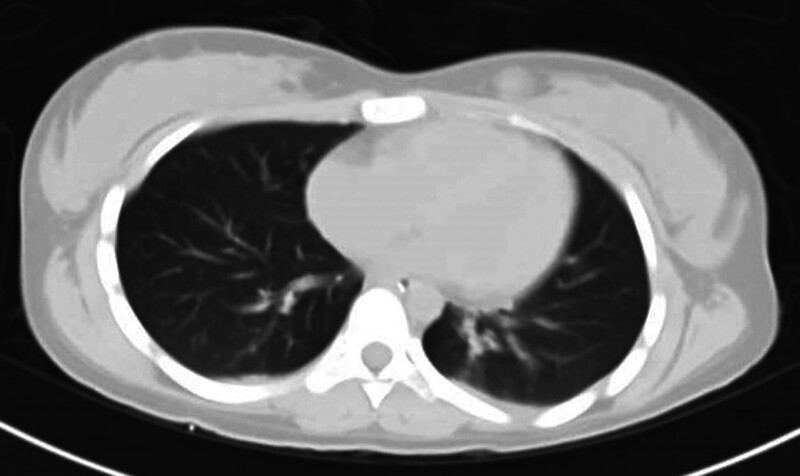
The chest CT scan after the patient developed a fever. The CT scan showed that she had pneumonia.
